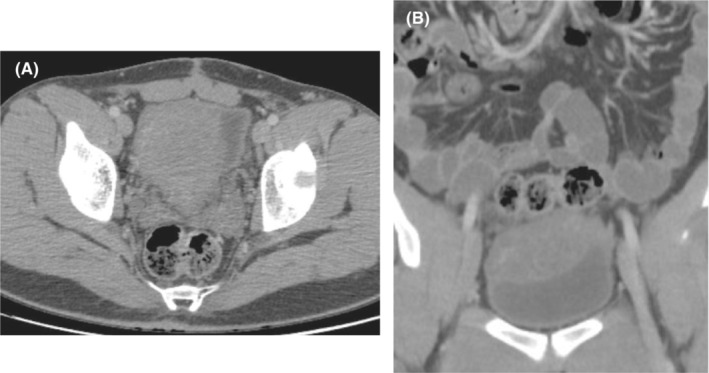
CT scan of the abdomen and pelvis. (A) Axial section showing a high attenuated lobular marginated mass occupying the bladder. Note the diffused thickening of the bladder wall. (B) Coronal section confirming aforementioned changes.
